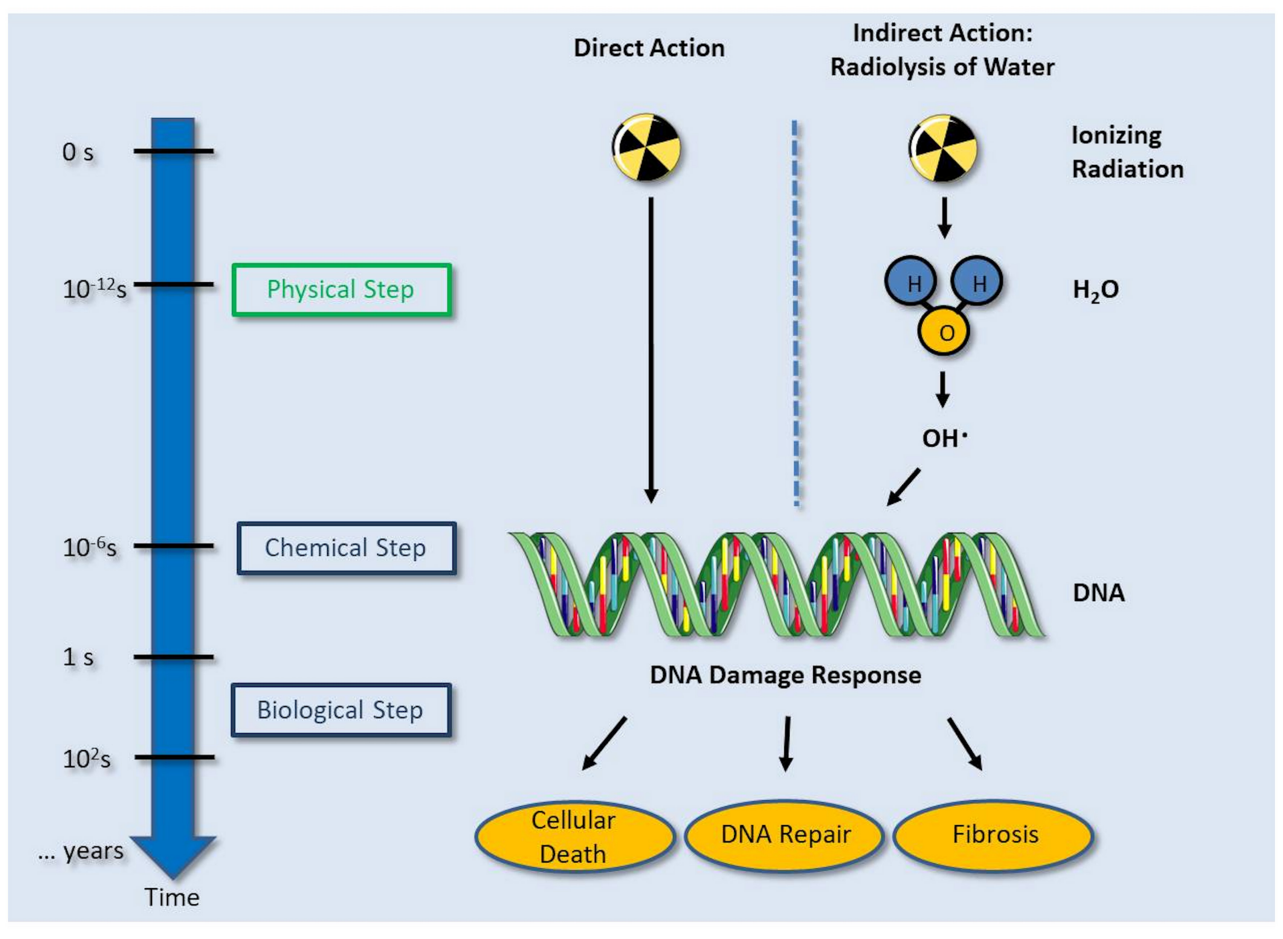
Biological effect of X-ray (ionizing radiation) on human tissue.
